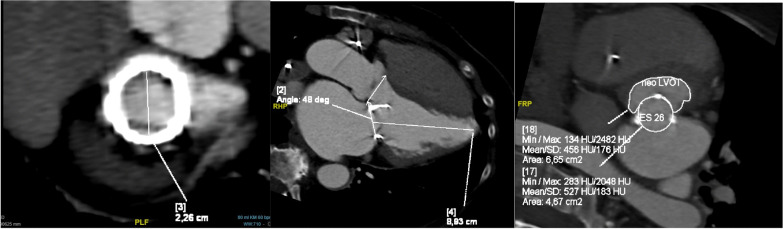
CT assessment of (1) internal diameter of the prosthesis, (2) aortomitral angel of 132°, (3) neo LVOT area of 6.6 cm^2^. LVOT: left ventricular out flow tract
